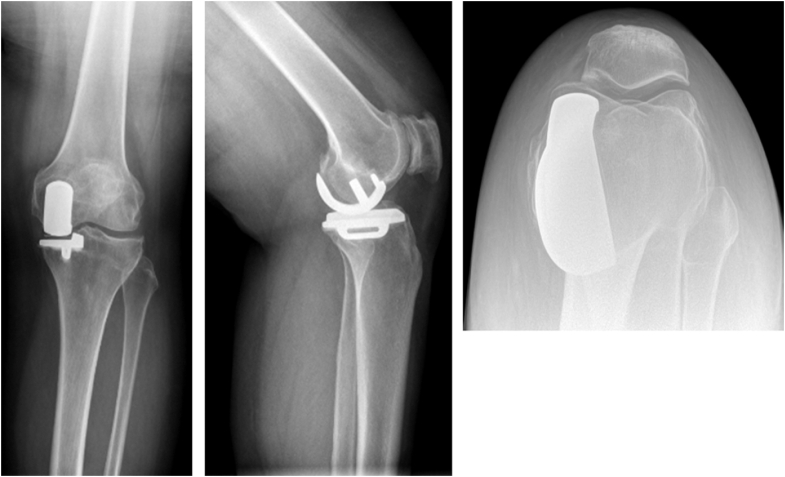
Anterior-posterior, lateral, and sunrise X-rays of the left UKA prior to revision.
